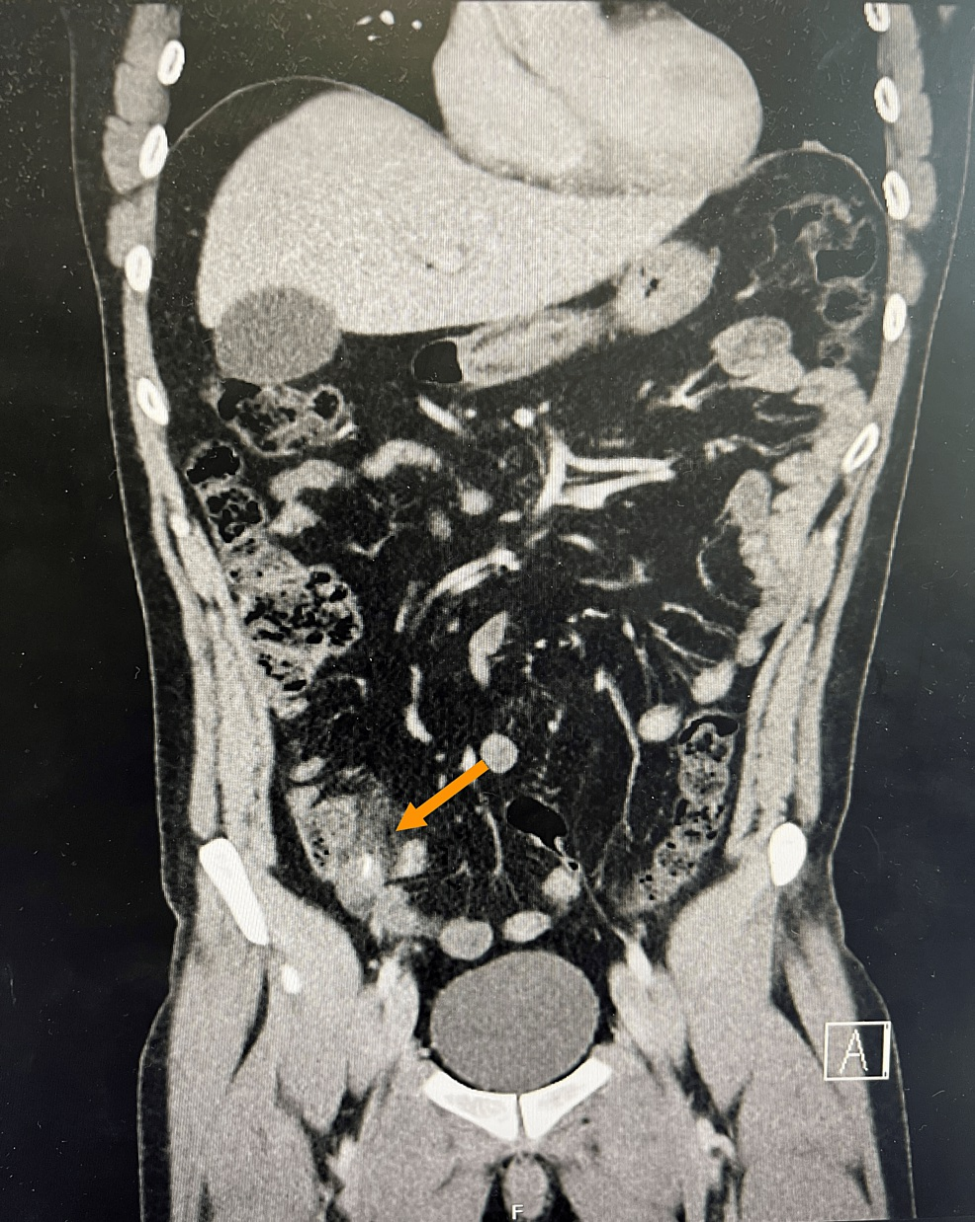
A coronal cut section of the CT abdomen and pelvis reveals an inflammatory condition and central calcification within a hollow viscus.
